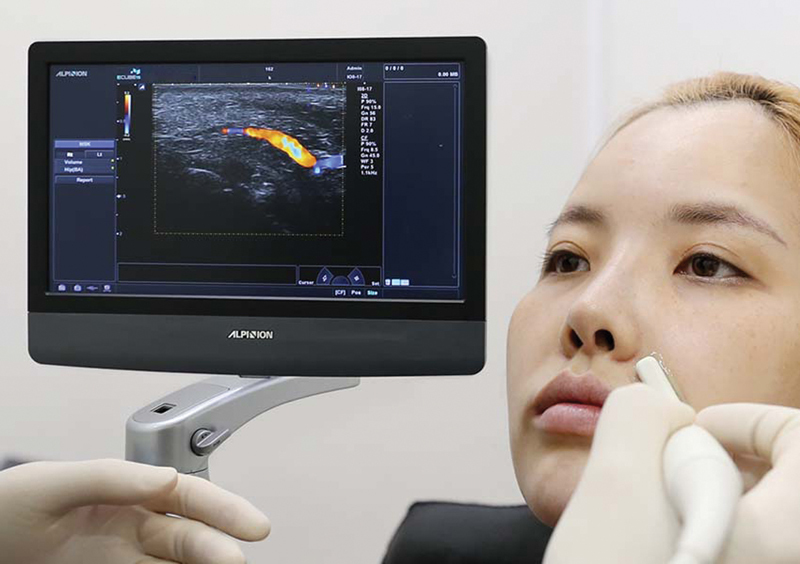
Doppler ultrasound probe being used at the nasolabial fold area. The facial artery is detected at the subcutaneous layer.
